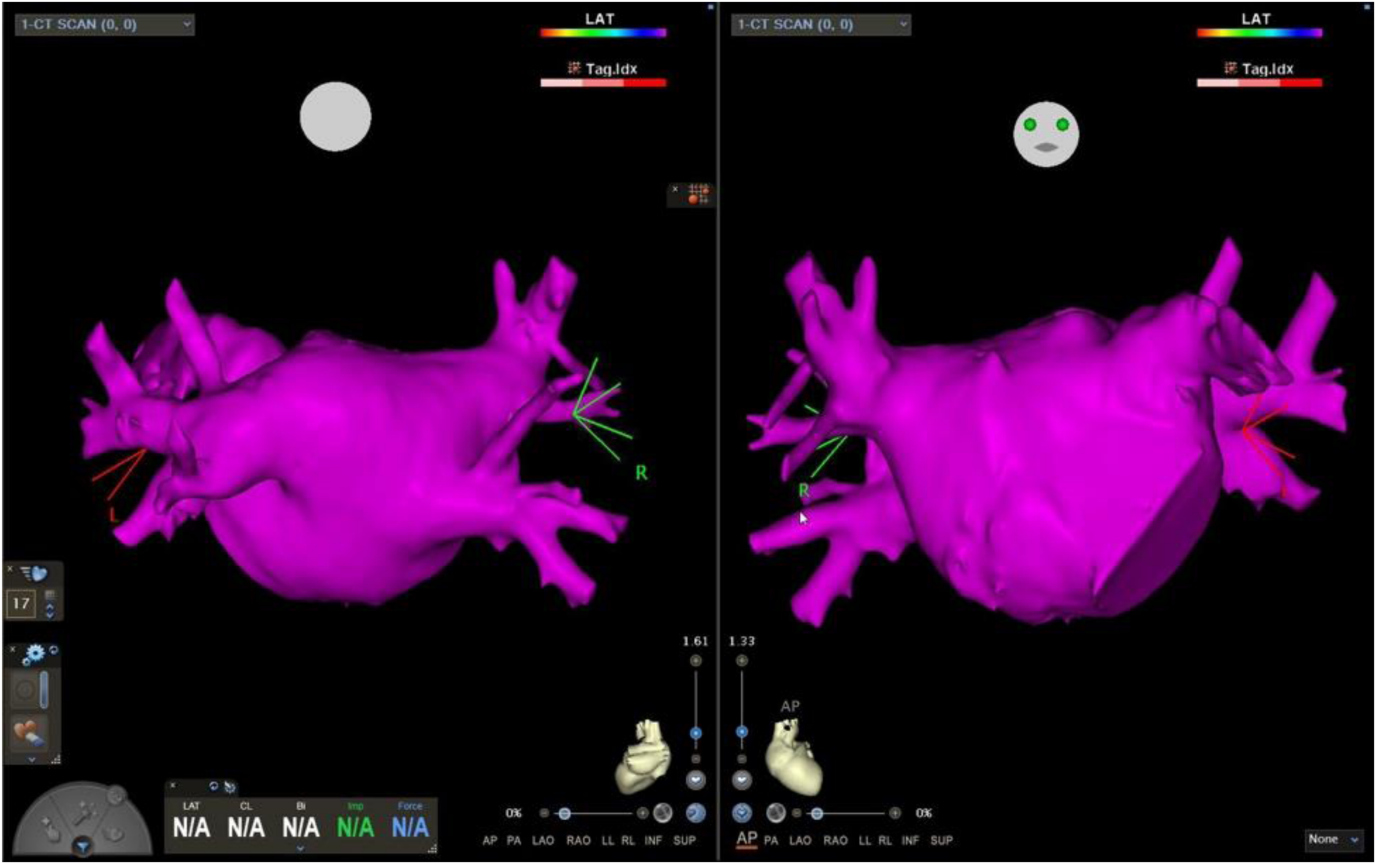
Left atrial CT scan segmentation. Cardiac computed tomography, with a left atrial volume of 70 ml and a left pulmonary venous drainage pattern R2a/L1.
